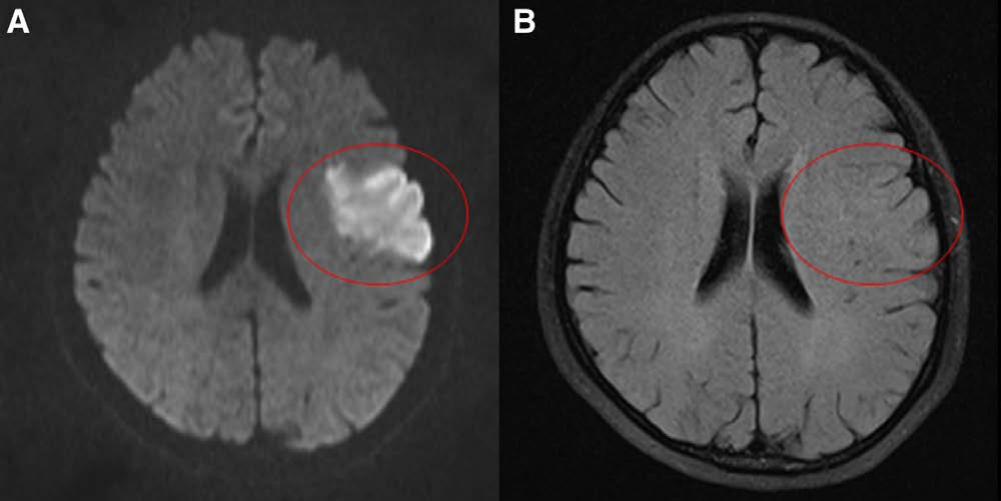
DWI showed high signal (A). FLAIR showed no obvious abnormalities (B). DWI = diffusion-weighted imaging, FLAIR = fluid-attenuated inversion recovery.
